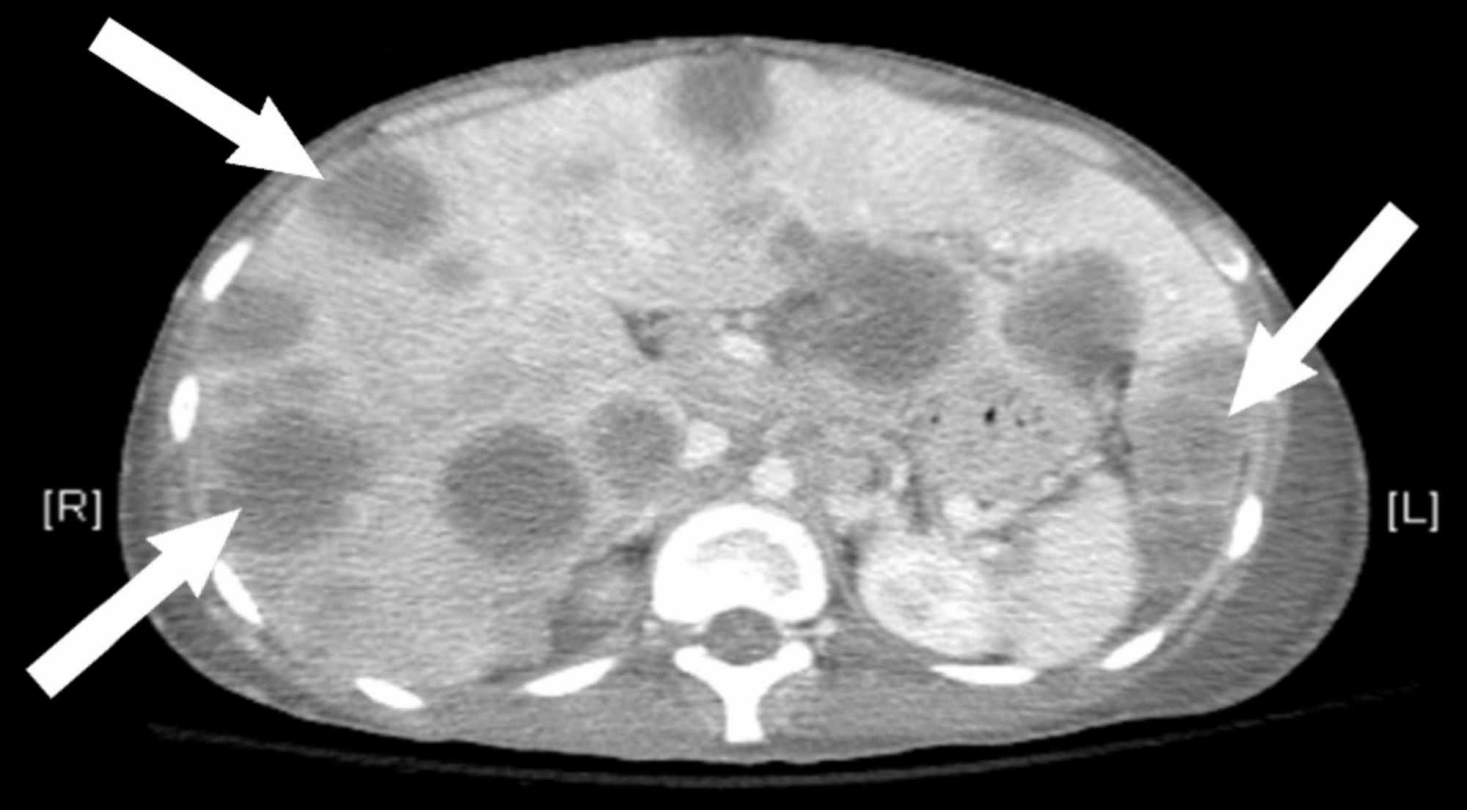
Axial CT scan of the abdomen with intravenous contrast demonstrating innumerable liver metastases. *Arrows demonstrating significant metastatic tumor burden in the liver with massive hepatomegaly.
